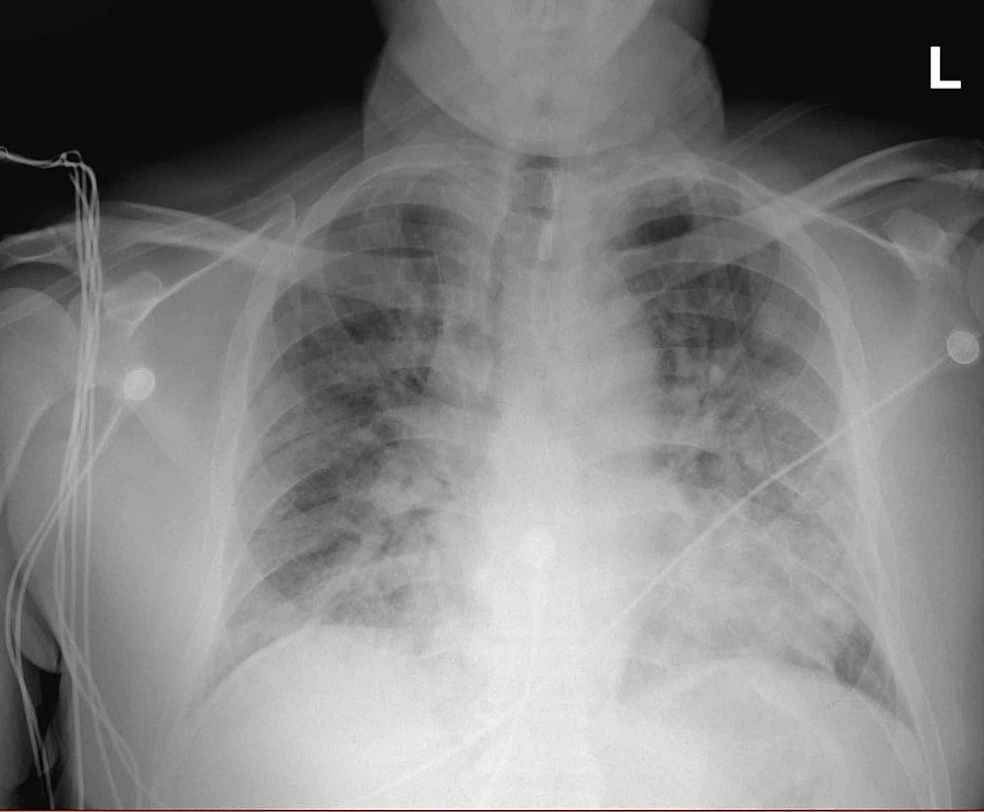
Chest X-ray of the patient The image shows moderate bilateral opacities without effusion
